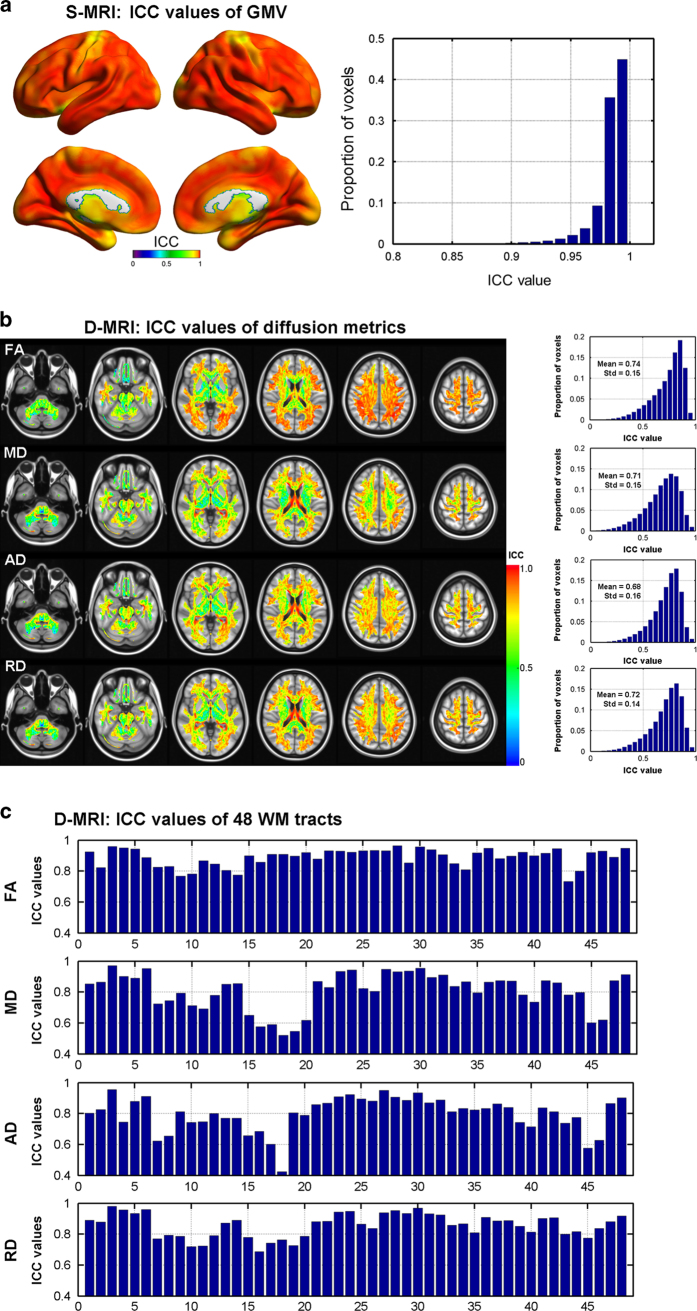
ICC values of GMV and diffusion metrics. (a) ICC values of GMV derived from S-MRI data. (b) ICC values of four diffusion metrics derived from D-MRI data. (c) ICC values of diffusion metrics in the 48 WM tracts of JHU ICBM DTI-81 atlas. The brain figures were visualized using the BrainNet Viewer (http://www.nitrc.org/projects/bnv/)^63^.
